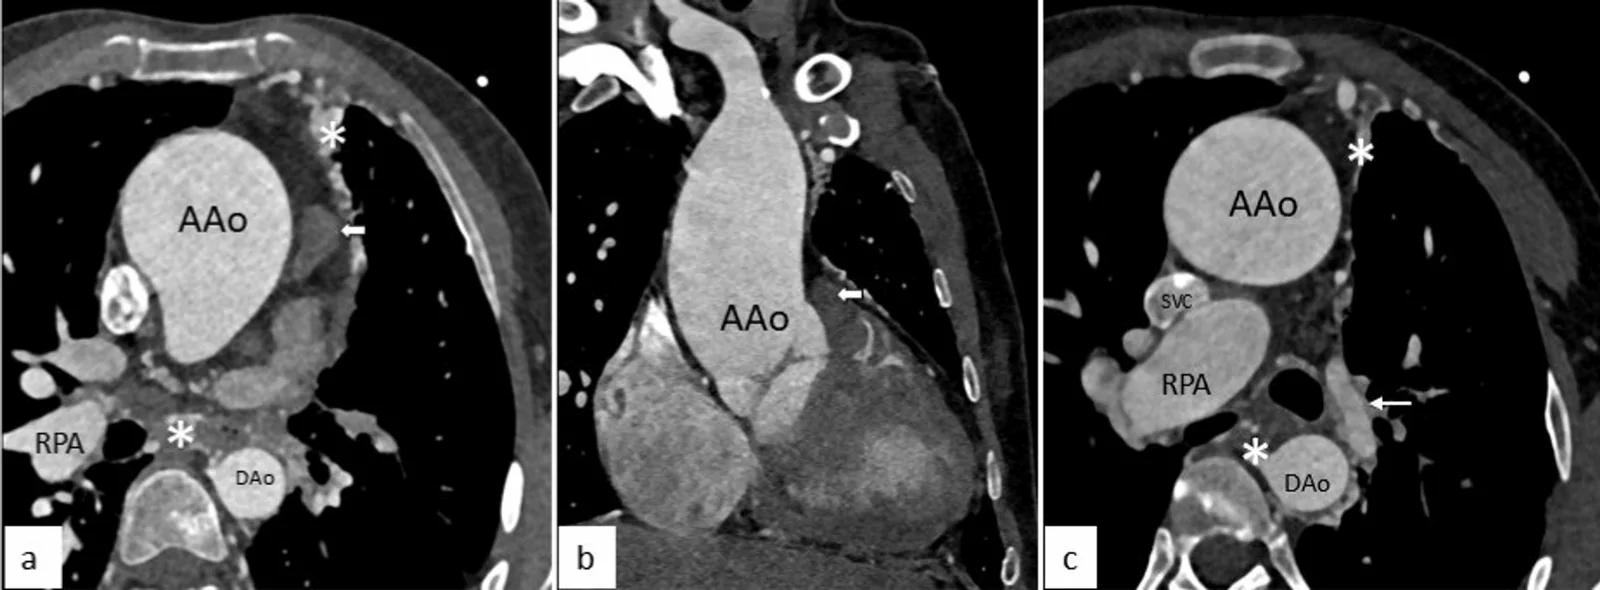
Cardiac CT demonstrating atretic MPA with reformed LPA (a) Multislice Cardiac CT Axial and (b) coronal image showing non-opacified atretic MPA (block arrow). Multiple mediastinal and peribronchial MAPCAs are seen(asterisk), (c) Cardiac CT Axial image, arrow showing reformed small caliber left pulmonary artery (LPA) in left hilum (white arrow) via multiple MAPCAs (stars). RPA- right pulmonary artery, AAo- Ascending Aorta, DAo- Descending Aorta, MPA- Main pulmonary artery, SVC- Superior Vena Cava.
